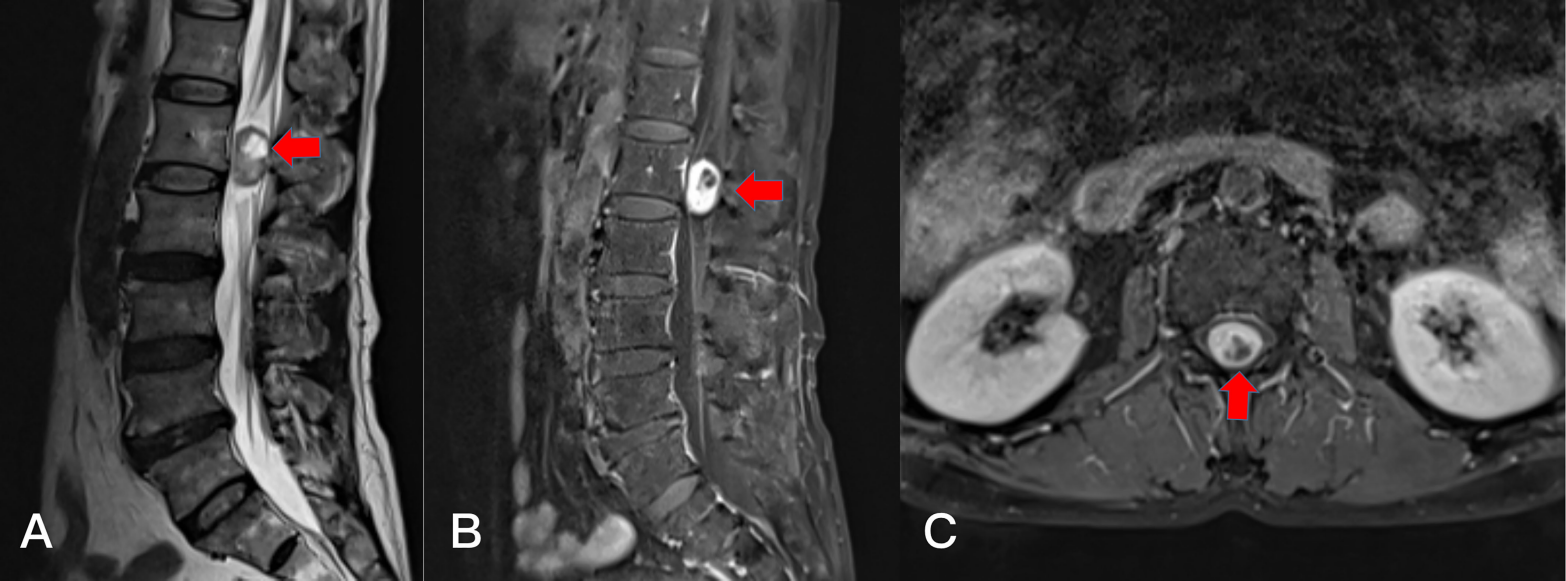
Preoperative T2-weighted MRI on sagittal (A) plane, T1-weighted enhanced sagittal (B) plane, and axial (C) planes, revealing a subdural tumor in the L1-2 segment (red arrows).
